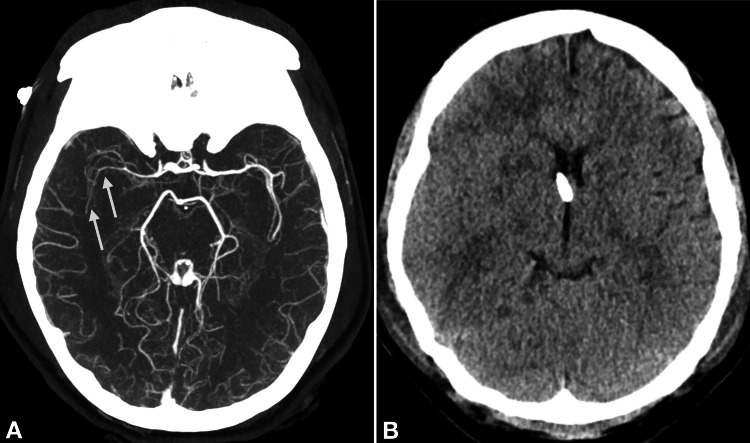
Second computed tomography scan performed 12 hours after initial imaging (A) Persistent vasospasm in the right middle cerebral artery on contrast computed tomography angiography (arrows). (B) Non-contrast CT shows progressive swelling of the right cerebral hemisphere; an external ventricular drain is visible.
